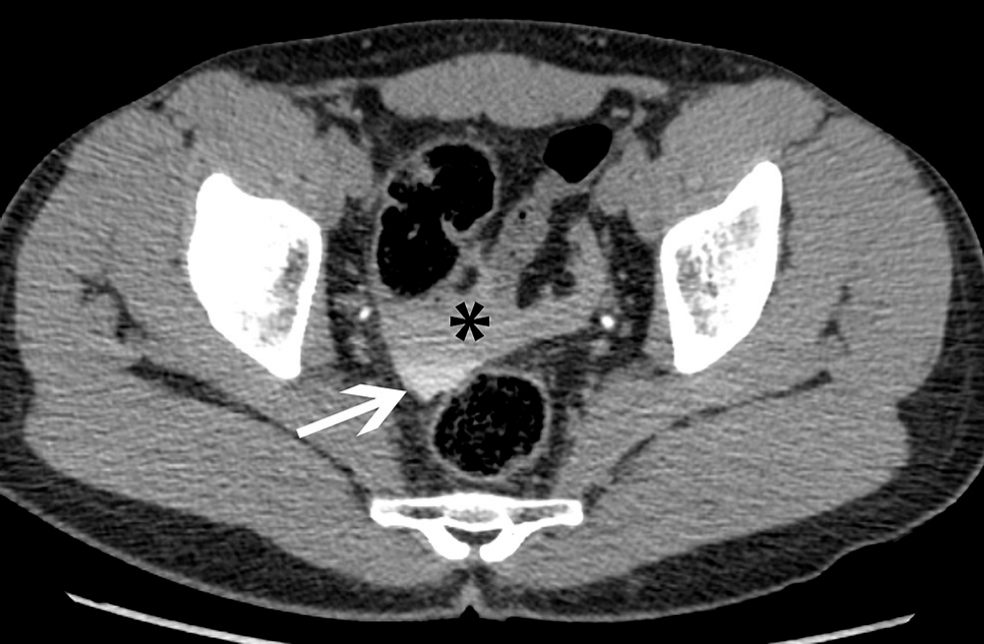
Axial CT image from delayed scan shows high attenuation fluid (black asterisk) in the pelvis representing extravasated contrast material. Note the higher attenuation portion layering dependently (white arrow).
